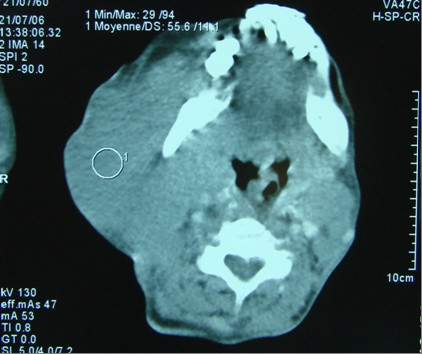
Computed tomography of the head and neck shows the oropharyngeal process and a bulky cervical mass.
